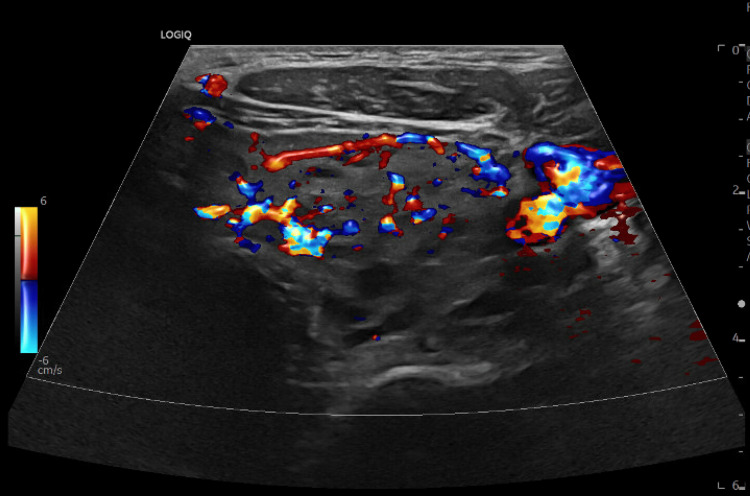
Ultrasound of the thyroid showing a diffusely heterogeneous thyroid with increased vascularity
